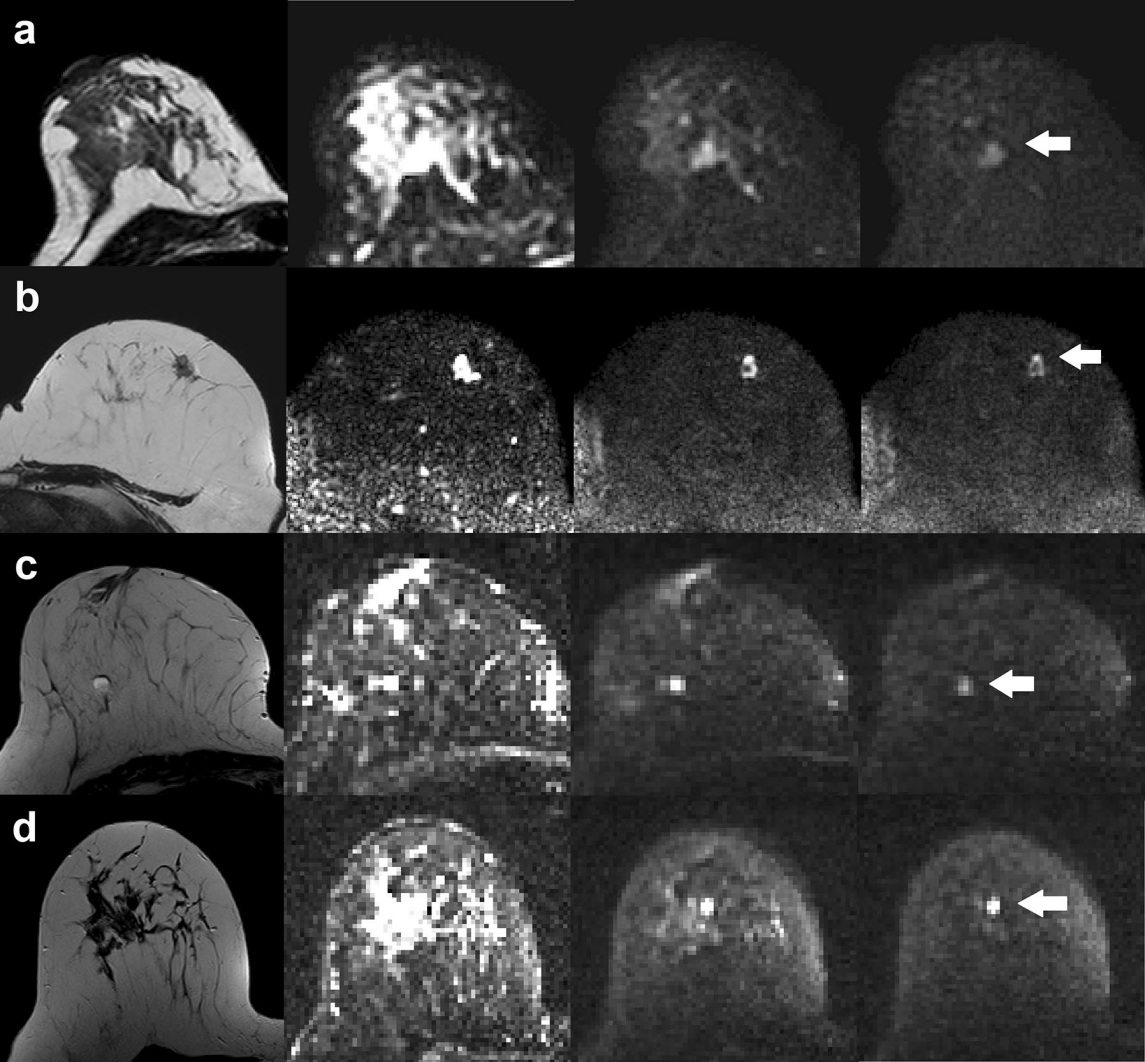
Examples of T2-weighted images (column on the left) and transversal DW images (*b*-values 0, 750 and 1500 s/mm^2^, respectively) of 4 patients. White arrows depict location of the lesions. (a) Benign lesion, cohort A. (b) Malignant lesion, cohort A. (c) Benign lesion, cohort B. (d) Malignant lesion, cohort B.
